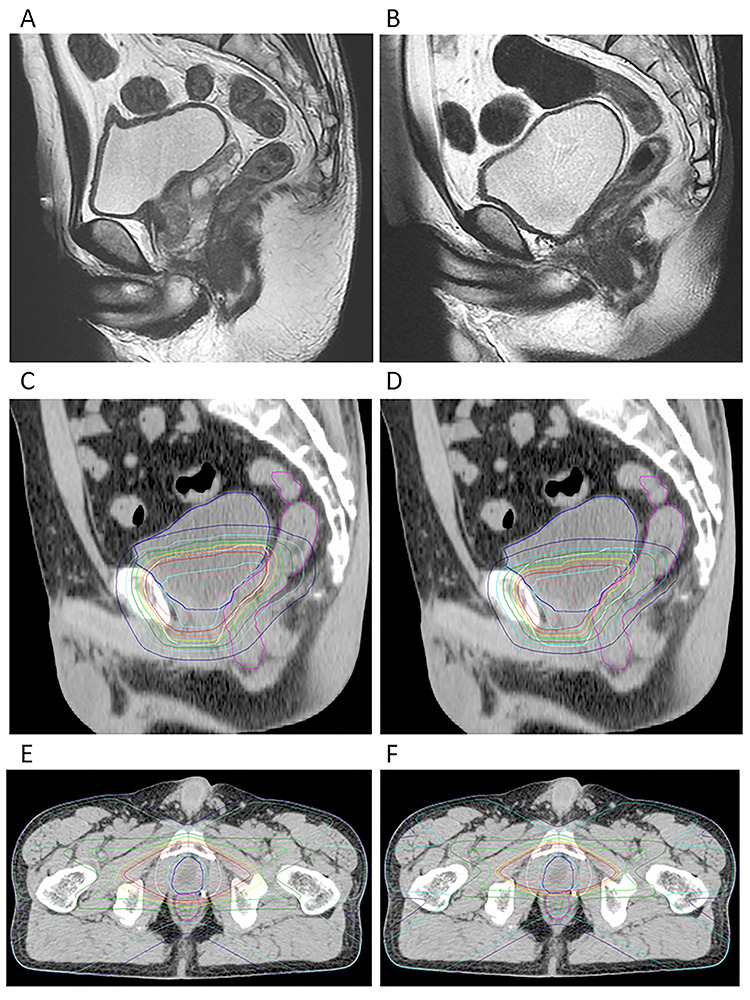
Representative dose distribution of hypofractionated salvage radiotherapy. MRI images before (A) and after (B) radical prostatectomy. Dose distributions: sagittal views of the initial (C) and boost (D) plans and axial views of the initial (E) and boost (F) plans (isodose lines: 95% in red; 90% in orange, 80% in yellow, 70% in yellow–green, 50% in green, 30% in light-blue, and 10% in blue).
